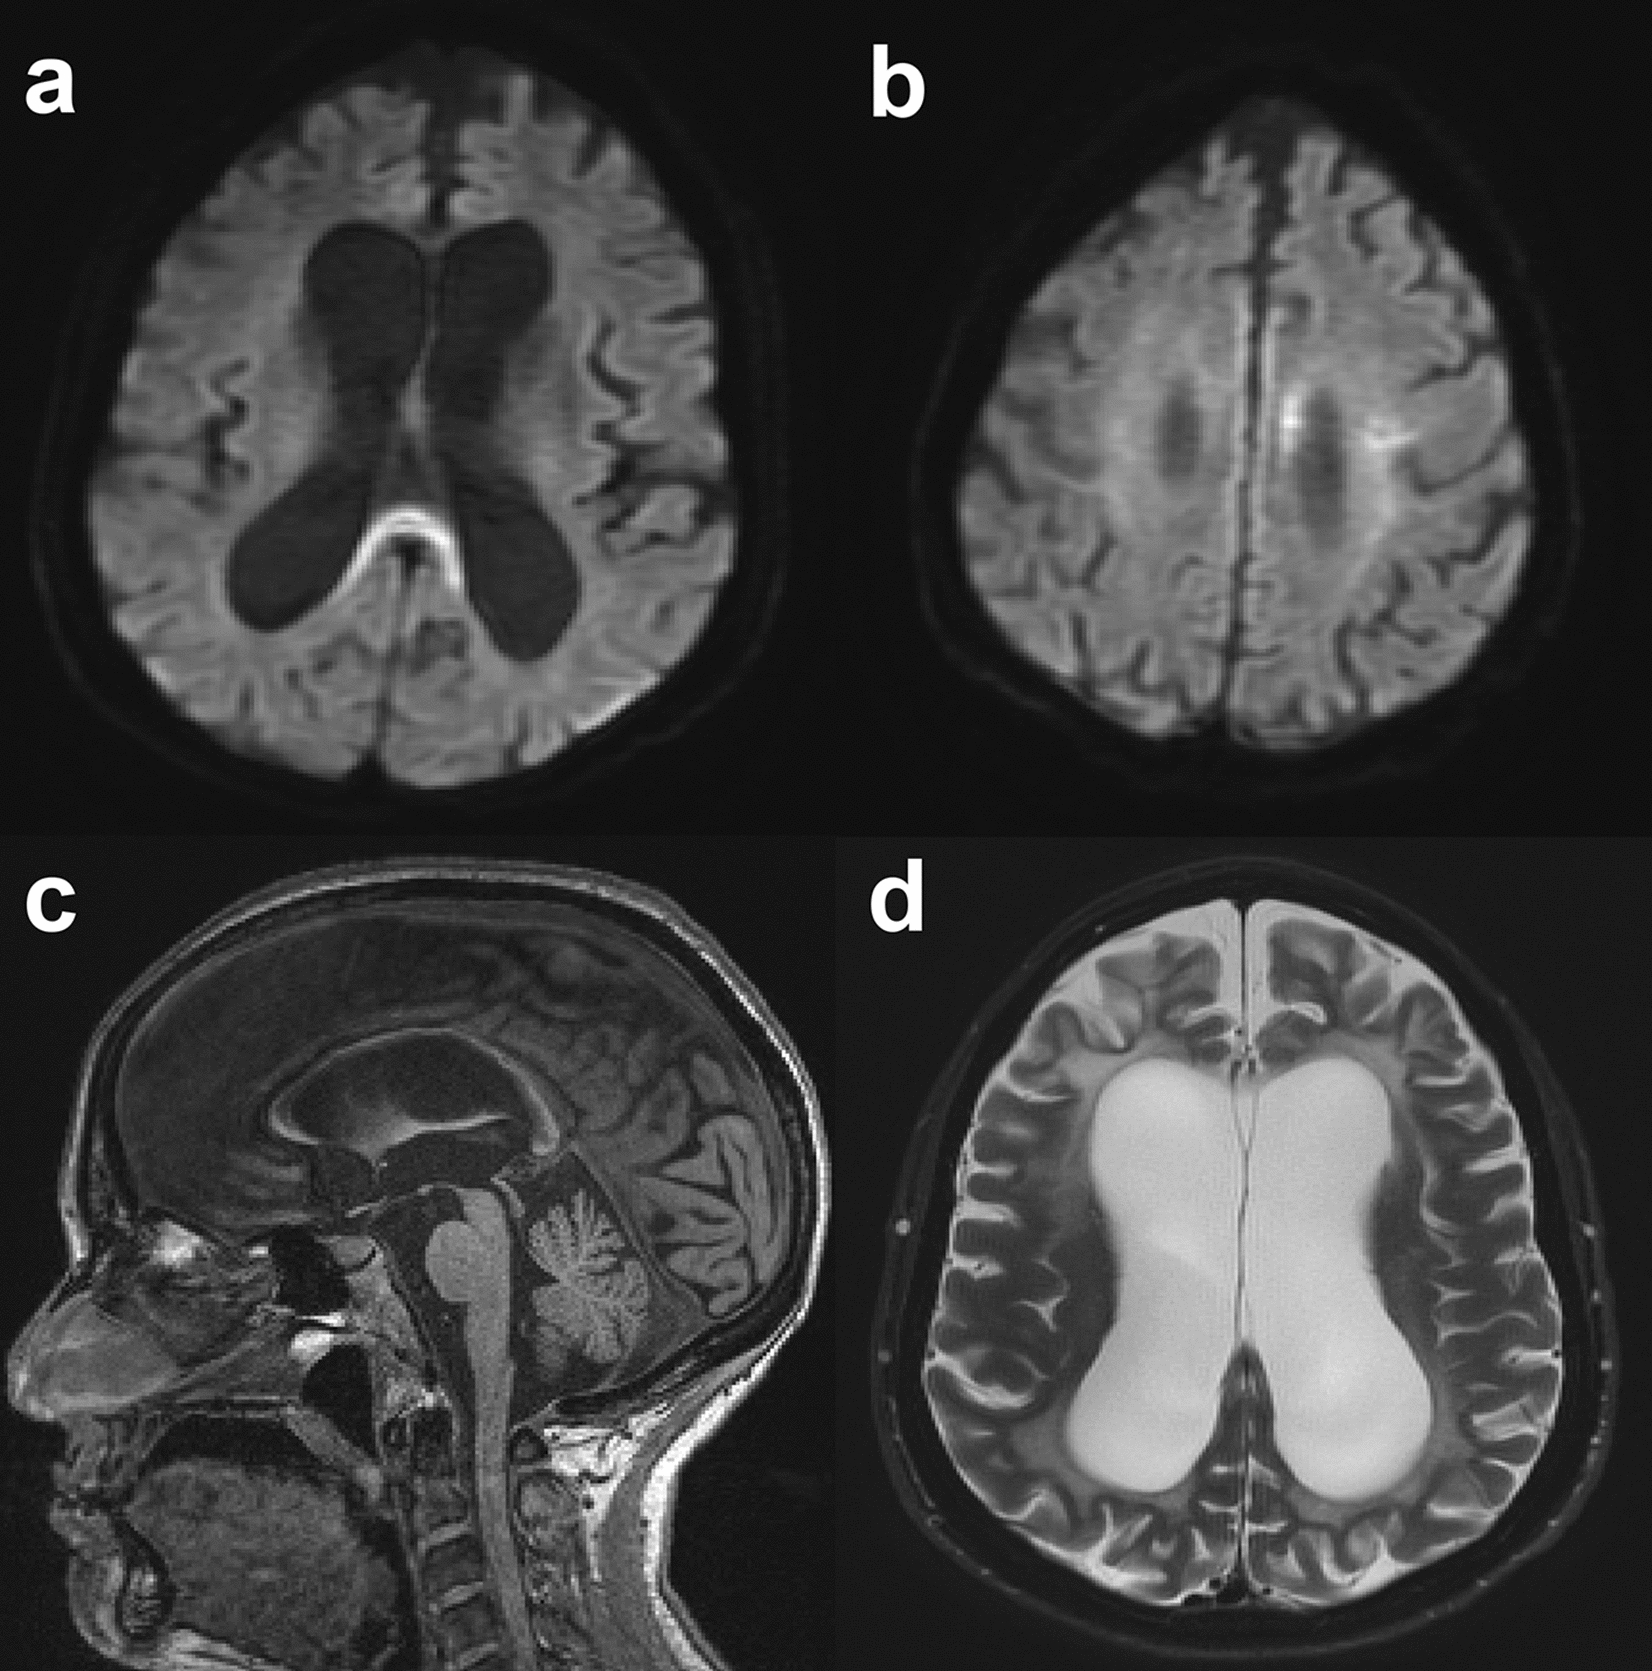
Brain MRI features of *CSF1R*-related leukoencephalopathy. The images were obtained from a 47-year-old female with progressive cognitive and behavioral deficits, and motor impairments with an approximate 3-year disease duration. Her father died of progressive dementia. The commercial genetic testing demonstrated the presence of heterozygote CSF1R mutation, p.L786S, c.2357 T > C. a, b Restricted diffusion in the splenium and left body of corpus callosum. c Thinning of corpus callosum on the sagittal section. d T2 hyperintensities in frontal and parietal white matter on the axial section. Courtesy of Daniel F. Broderick, M.D., Mayo Clinic Florida
